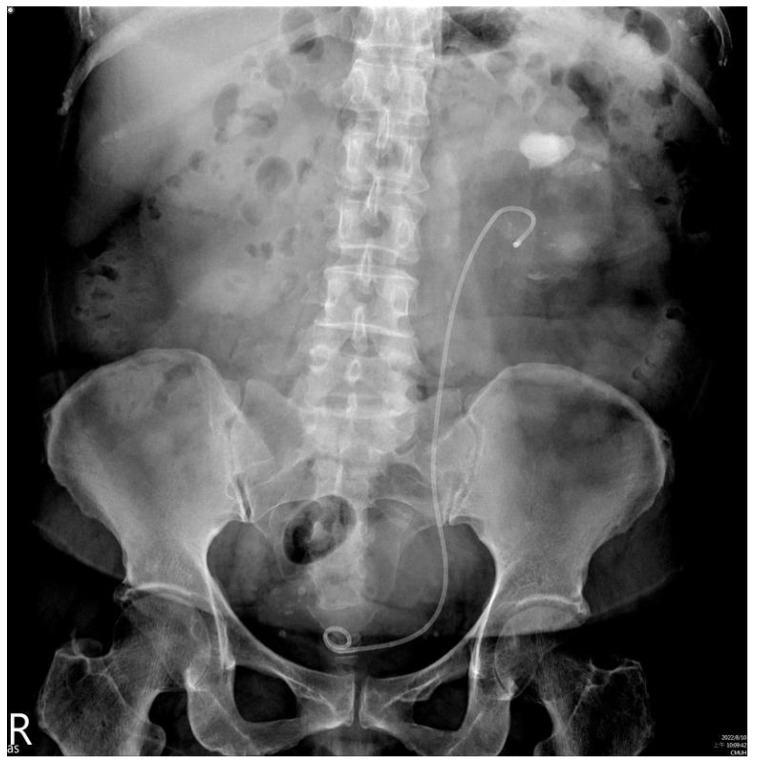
Postoperative findings of a kidney, ureter and bladder radiograph suggest a residual stone about 24 mm in size.
